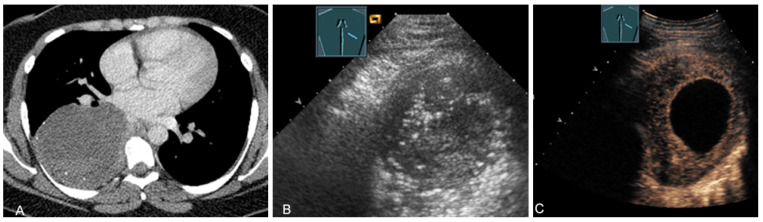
A young patient with tumor formation in the paravertebral mediastinum on CT (provided by Prof. Dr. Andreas H. Mahnken, Marburg, Germany) (A), B-mode US (B), and CEUS (C), surgically confirmed as ganglioneuroma.
